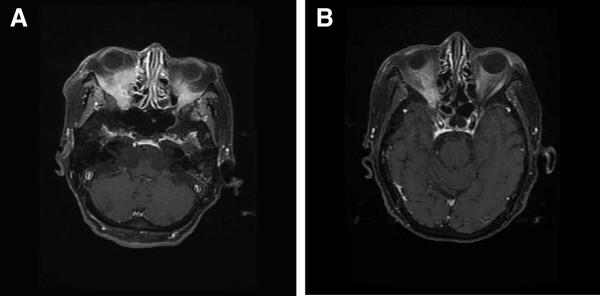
T1-weighted magnetic resonance images of the orbit with gadolinium infusion and fat suppression. (A) Coronal image taken at initial presentation showing a mass involving the right orbital space and extending into the ethmoidal sinus. The mass is displacing the globe. A small mass is also shown in the left orbital space. (B) Coronal image showing reduction of the pseudotumor 12 weeks after treatment.
